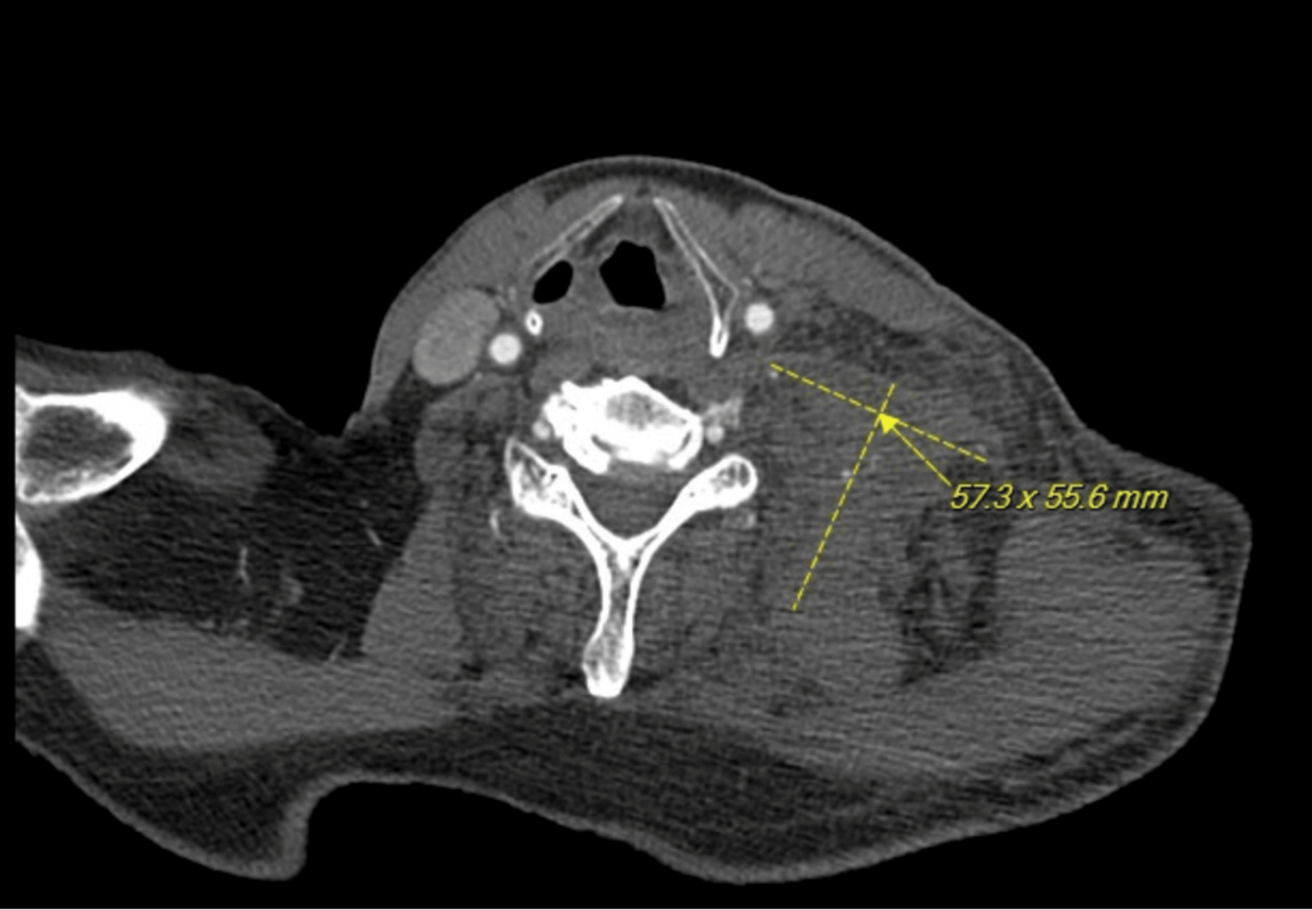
Computed tomography angiography (CTA) of the neck with and without contrast. This CTA demonstrates foci of high-density material within the hematoma (margins indicated by dotted lines) favored to reflect contrast extravasation (indicated by the yellow arrow).
